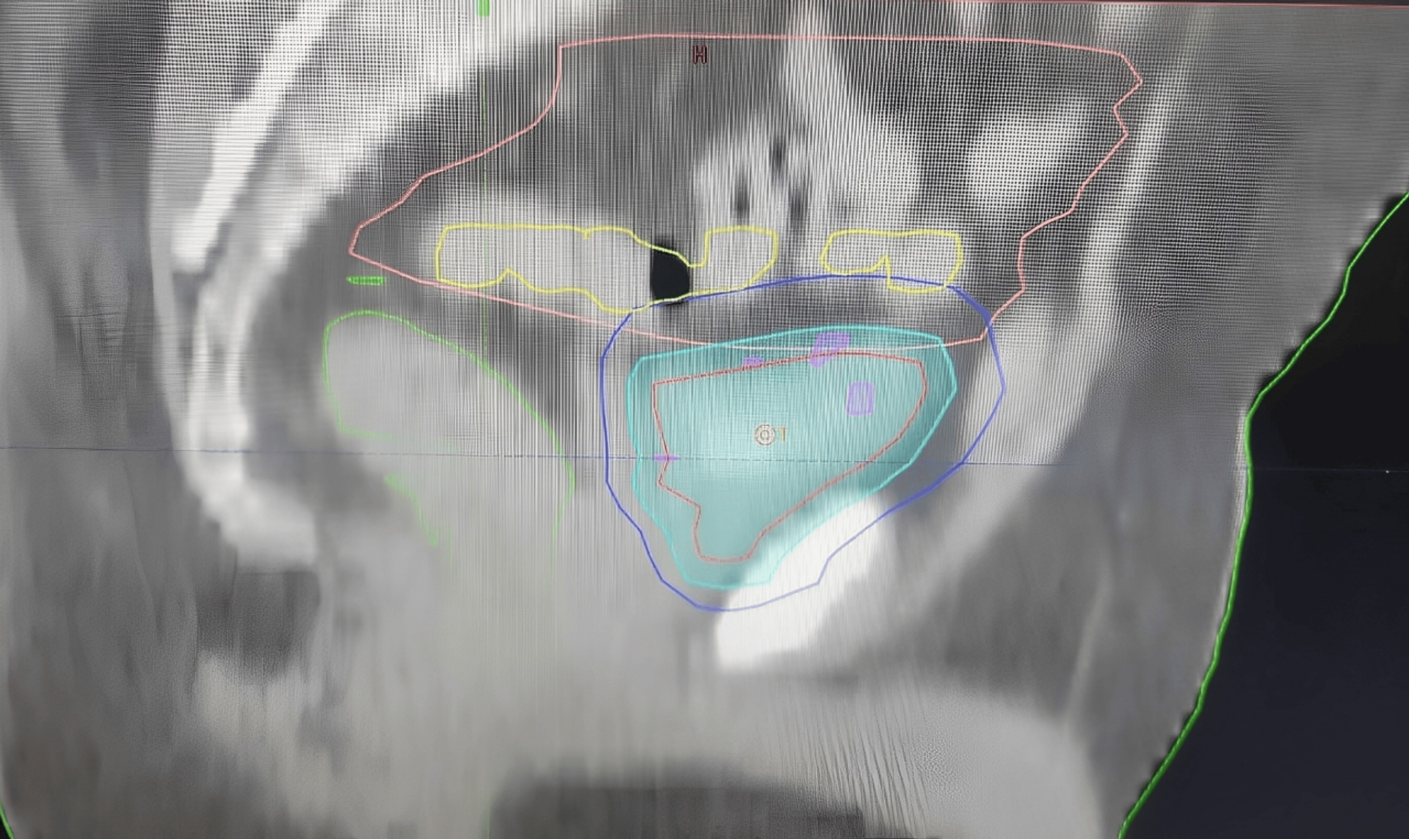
Planning CT sagittal view with an empty bladder, CTV, small PTV, and large PTV red: whole bladder; cyan blue: CTV; dark blue: PTV; pink: bowel bag; yellow: colon; green: rectum CT: computed tomography; CTV: clinical target volume; PTV: planning target volume
